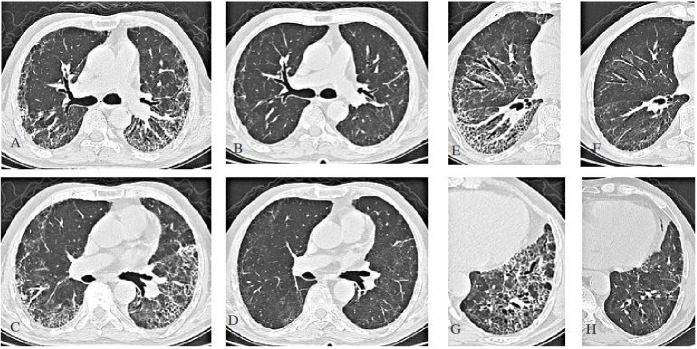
a-c-e-g: Chest CT of the patient who presented with dyspnea at 13 weeks post-COVID-19. b-d-f-h: Significant regression in CT findings of the patient are observed at the 25th week after steroid treatment. (From archives of Aydın Yılmaz)
